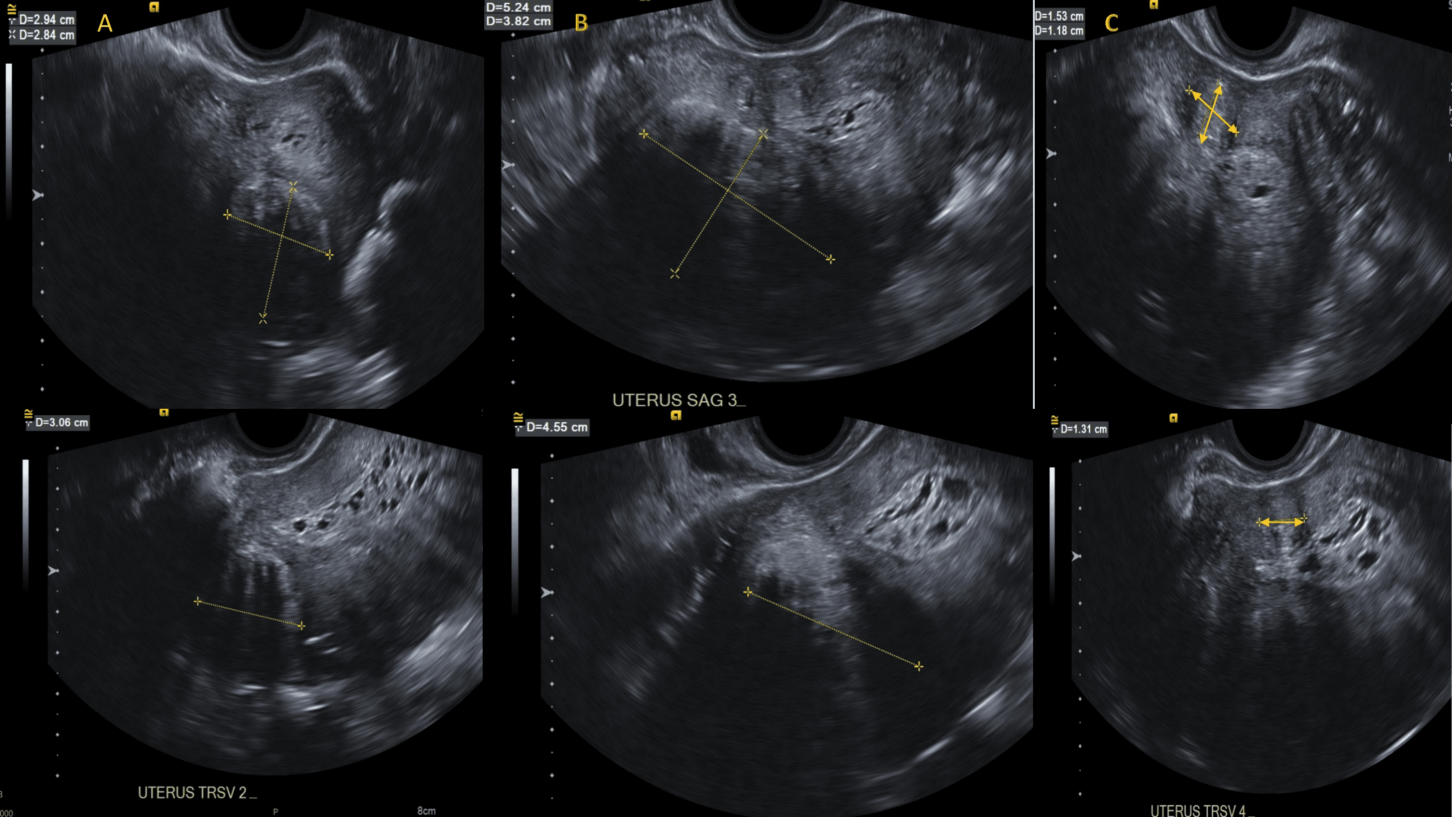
Several different-sized myometrial lumps located in various places on the uterus were visible on a transvaginal ultrasound.
